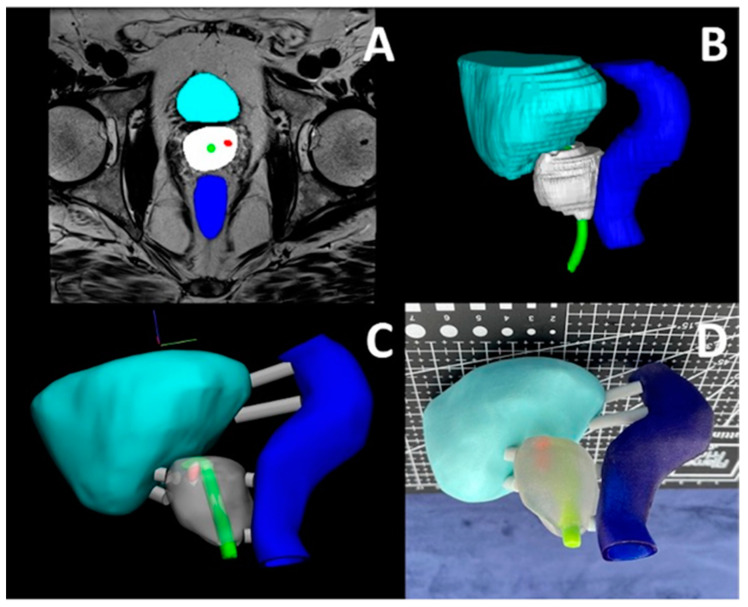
Full color MJT 3DP anatomic model for prostate cancer cryoablation planning. The segmented anatomy included prostate (white), urethra (green), bladder (cyan), rectum (blue) and lesion (red). (A) The axial MRI scan with segmented masks. (B) 3D visualization of the segmented masks. The MRI slice is visible due to the relatively larger slice thickness. (C) The smoothed digital 3D model in CAD software with the urethra and lesion visible through the transparent prostate, and (D) the final full color 3D printed using MJT technology.
